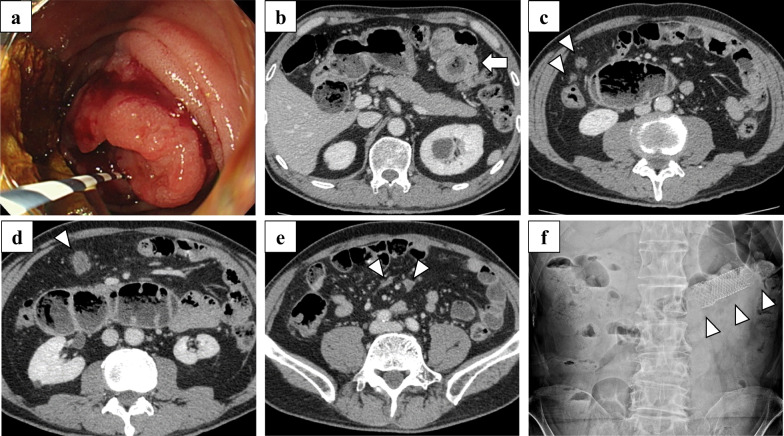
Images before chemotherapy. a Jejunal examination with an endoscope detected a circumferential hemorrhagic tumor in the upper jejunum. b Computed tomography revealed a tumor of the jejunum (arrow). c–e Multiple peritoneal metastases were observed spanning from the epigastric region down to the lower abdominal area (arrowheads). The larger peritoneal metastases were primarily located in the greater omentum (c, d). f An expandable metallic stent (Boston WallFlex^®^ Duodenal Stent 22 × 60 mm, arrowheads) was implanted and expanded
